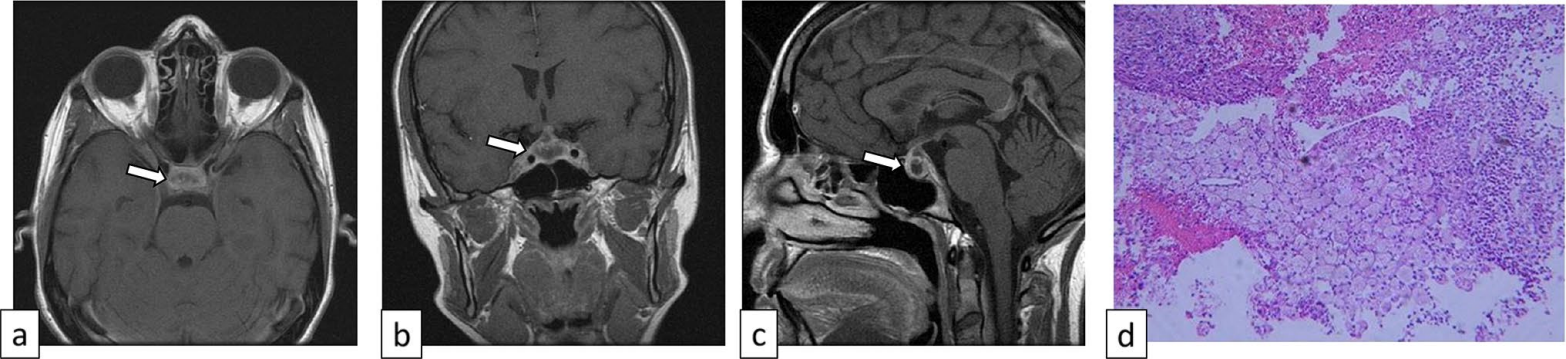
A 31-year-old woman with xanthomatous hypophysitis. Post-contrast T1-weighted images show an enlarged pituitary gland with central non-enhancing components and peripheral contrast enhancement (a–c, arrows). Pathology shows fragments of intact, normal pituitary gland infiltrated by foamy histiocytes (d). The images were cited and modified from [19]
